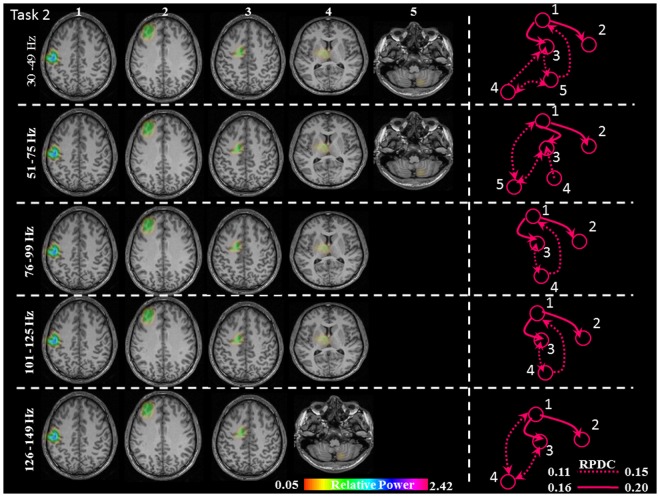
Relative gamma synchronization at the onset of the brisk pressing, Task 2. Results of the source and directionality analysis in the five frequency bands. The individual maps of power were spatially normalized and interpolated on standard T1-weighted MRI scans with 1 mm spacing in MNI space. The color bar represents the relative power. 1: primary sensorimotor cortex (S1M1); 2: dorsolateral prefrontal cortex (dPFC); 3: supplementary motor area (SMA); 4/5: thalamus (TH)/cerebellum (CER); RPDC, renormalized partial directed coherence.
